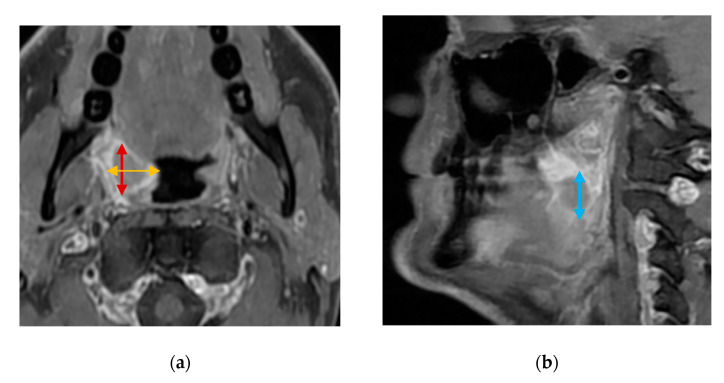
Contrast-enhanced, T1-weighted MRI images of a stage T1 squamous cell carcinoma of the right palatine tonsil: (a) axial orientation showing the mediolateral (yellow bi-directional arrows) and anteroposterior (red bi-directional arrows) diameters of the tumor; (b) sagittal orientation showing the craniocaudal (blue bi-directional arrows) diameter of the tumor.
